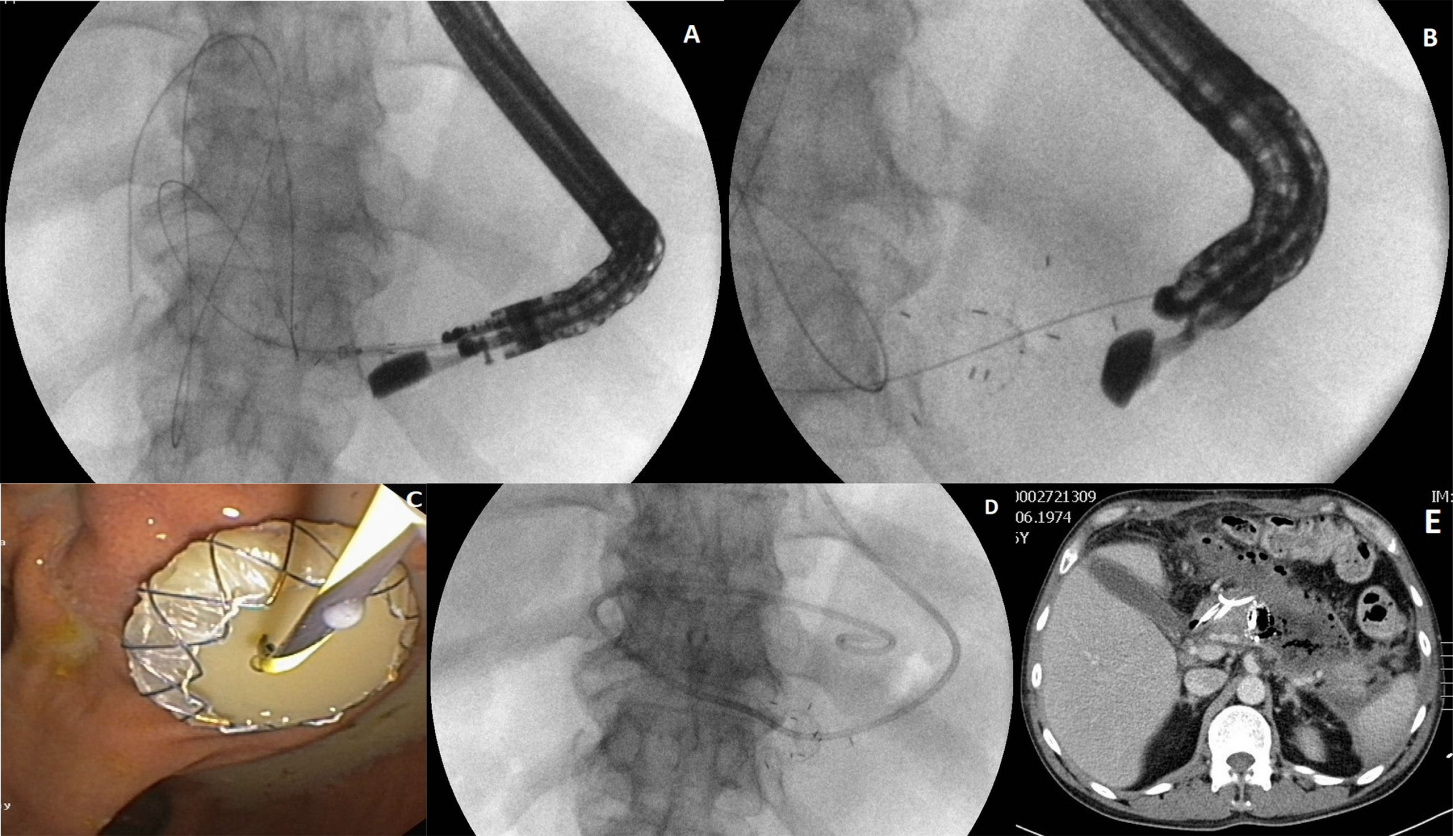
(A–E) Active (with flushing through nasocystic drainage) transmural drainage of a walled-off pancreatic necrosis. After the transmural fistula is created and a self-expanding stent (LAMS) is inserted transmurally (A–C) through the fistula, a nasal drain (D) is introduced along a guidewire into the necrotic area. Active transmural drainage of pancreatic necrosis is visible in computed tomography of abdomen (E).
